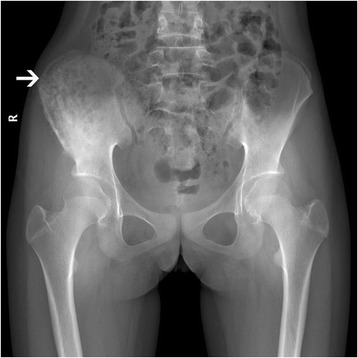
Anteroposterior radiograph of pelvis shows a predominantly lytic process with sclerosis and periosteal proliferation in the right iliac wing (*white arrow*)
